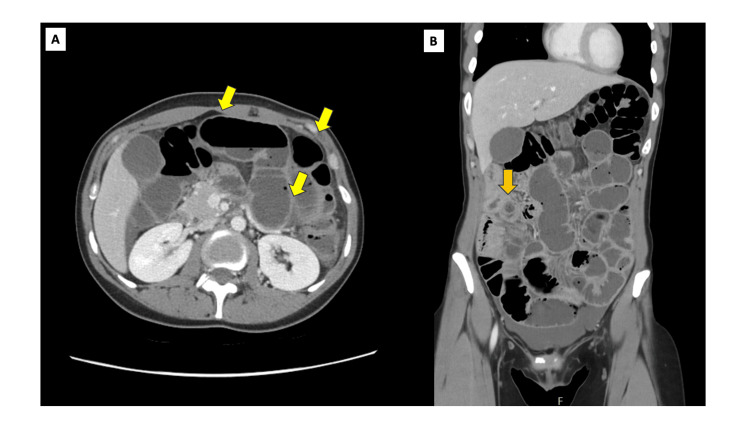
Case 2: CT images of small bowel obstruction. (A) Axial CT image demonstrating multiple dilated small bowel loops (yellow arrows), consistent with small bowel obstruction. (B) Coronal CT reconstruction confirming small bowel obstruction. On retrospective review, a subtle concentric configuration (orange arrow) may correspond to the site of intussusception. CT: computed tomography
